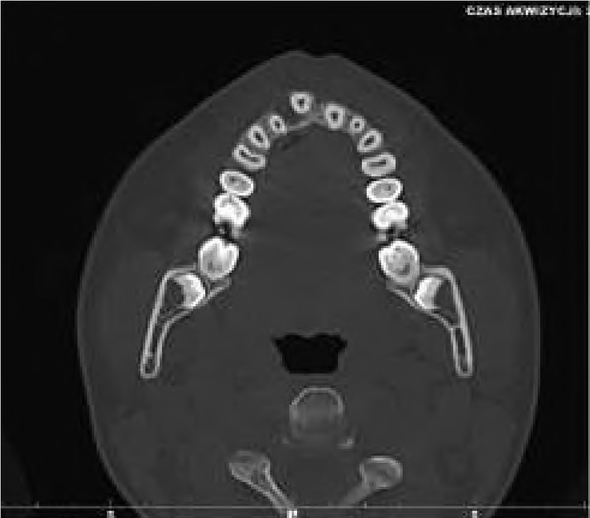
CT scan, osteolytic defect in the alveolar process. Ryc. 4. Skan TK. Zniszczenie struktury kostnej wyrostka zębodołowego.
